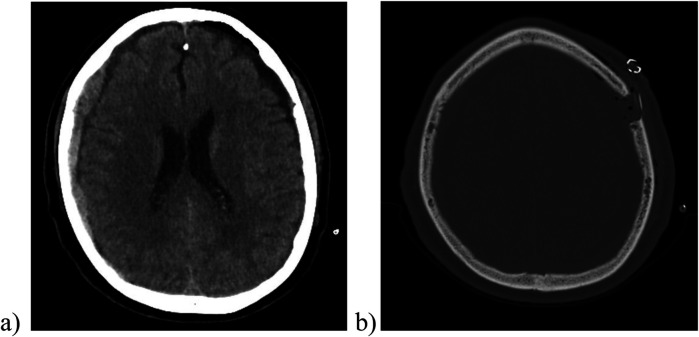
The postoperative cranial CT scan (image a, soft tissue window) showed a significant reduction in the size of the left-sided hematoma and the associated midline shift. In the image (b) (bone window) one can observe the placement of the subperiosteal/subgaleal drainage (no contact to the underlying brain tissue).
